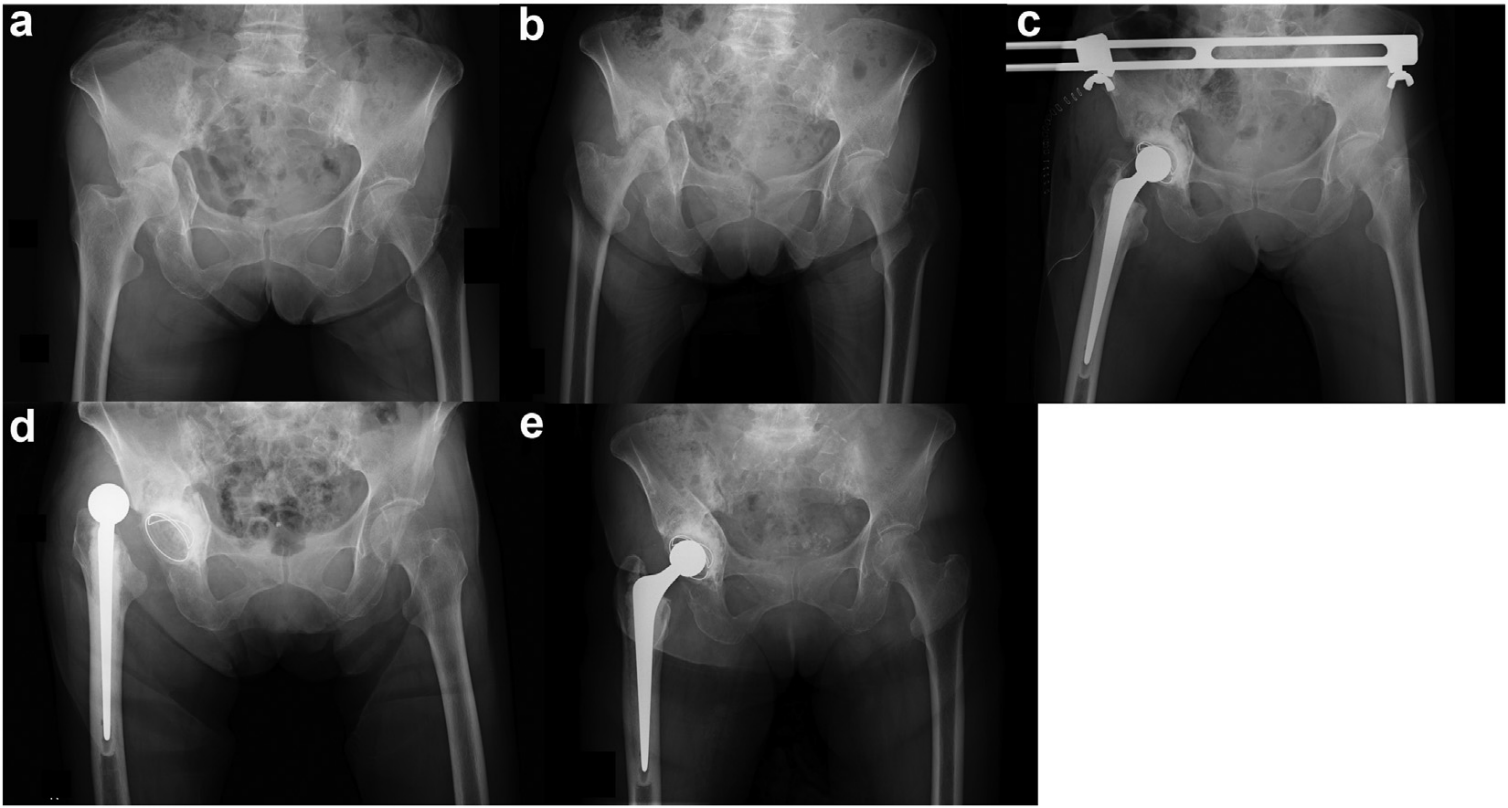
A case presentation (case 3). (a) The radiographic imaging at the patient’s first visit to our hospital showed a comminuted acetabular fracture with quadrilateral surface destruction as well as a femoral neck fracture. Good callus formation was evident on the acetabulum. (b) Radiographic imaging 2 months after the patient’s initial injury showed good callus formation. (c) Radiographic imaging after late THA with IBG 2 months after the initial trauma. (d) The patient experienced an anterior dislocation due to hyperextension at 2 months postoperatively. (e) Radiographic imaging at the 3-year follow-up showed no component loosening or any subsidence of the acetabular cemented cup.
